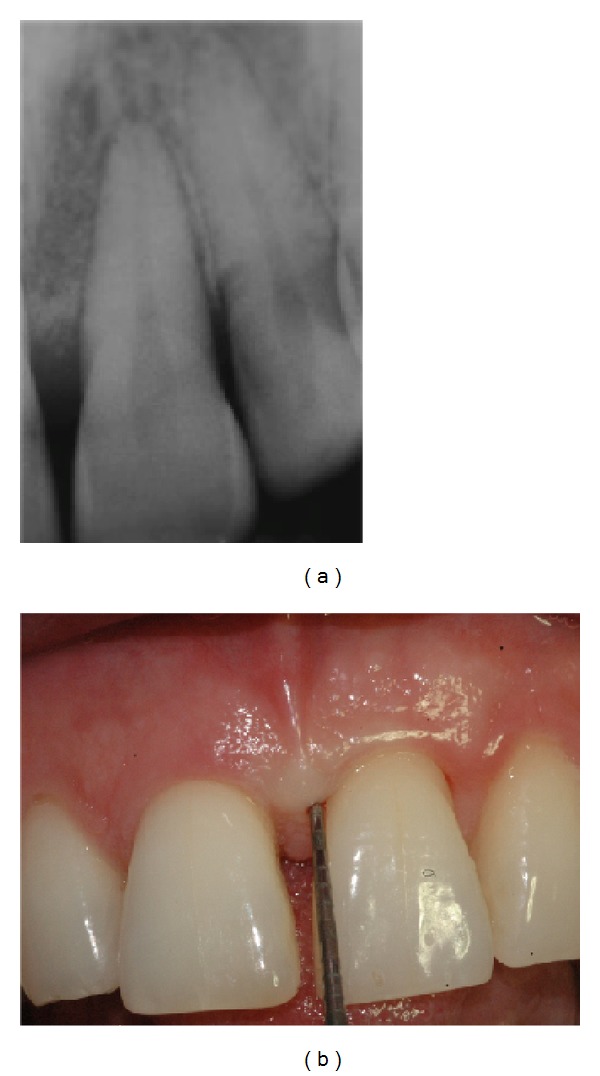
(a) X-ray at 24 months. (b) Clinical aspect showing attachment gain.
